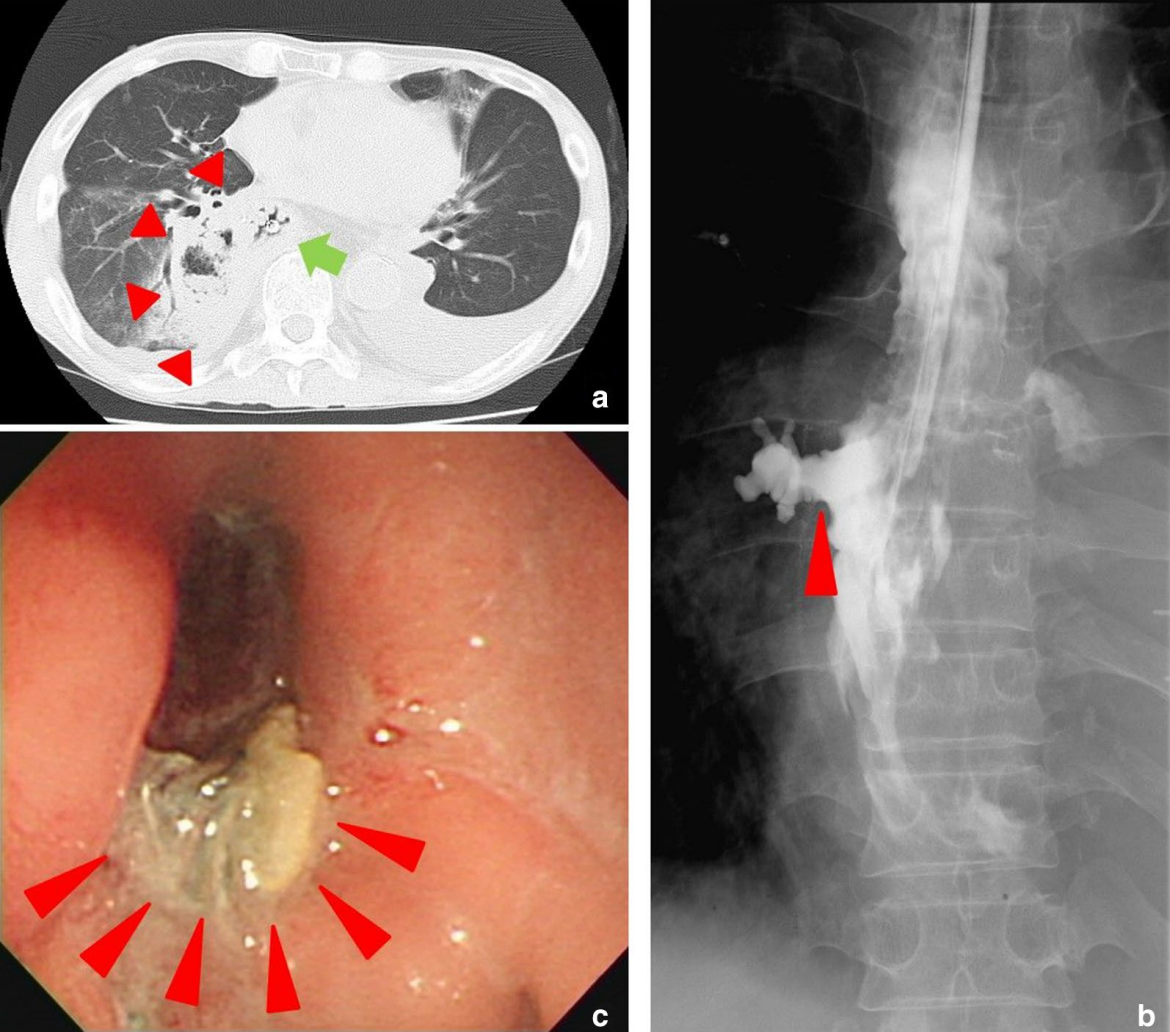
Imaging examinations of the fistula and pulmonary abscess performed before the conservative treatment. a A CT image showed a cavity with an air bubble on the right side of the gastric staple line. The green arrow indicates the gastric tube, and the red arrowheads show the abscess. b A Gastrografin esophagram showed extravasation into the right side of the thoracic cavity (red arrowheads). c An endoscopic examination confirmed that the fistula was located on the anal side of the anastomotic site. The red arrowheads indicate the fistula
